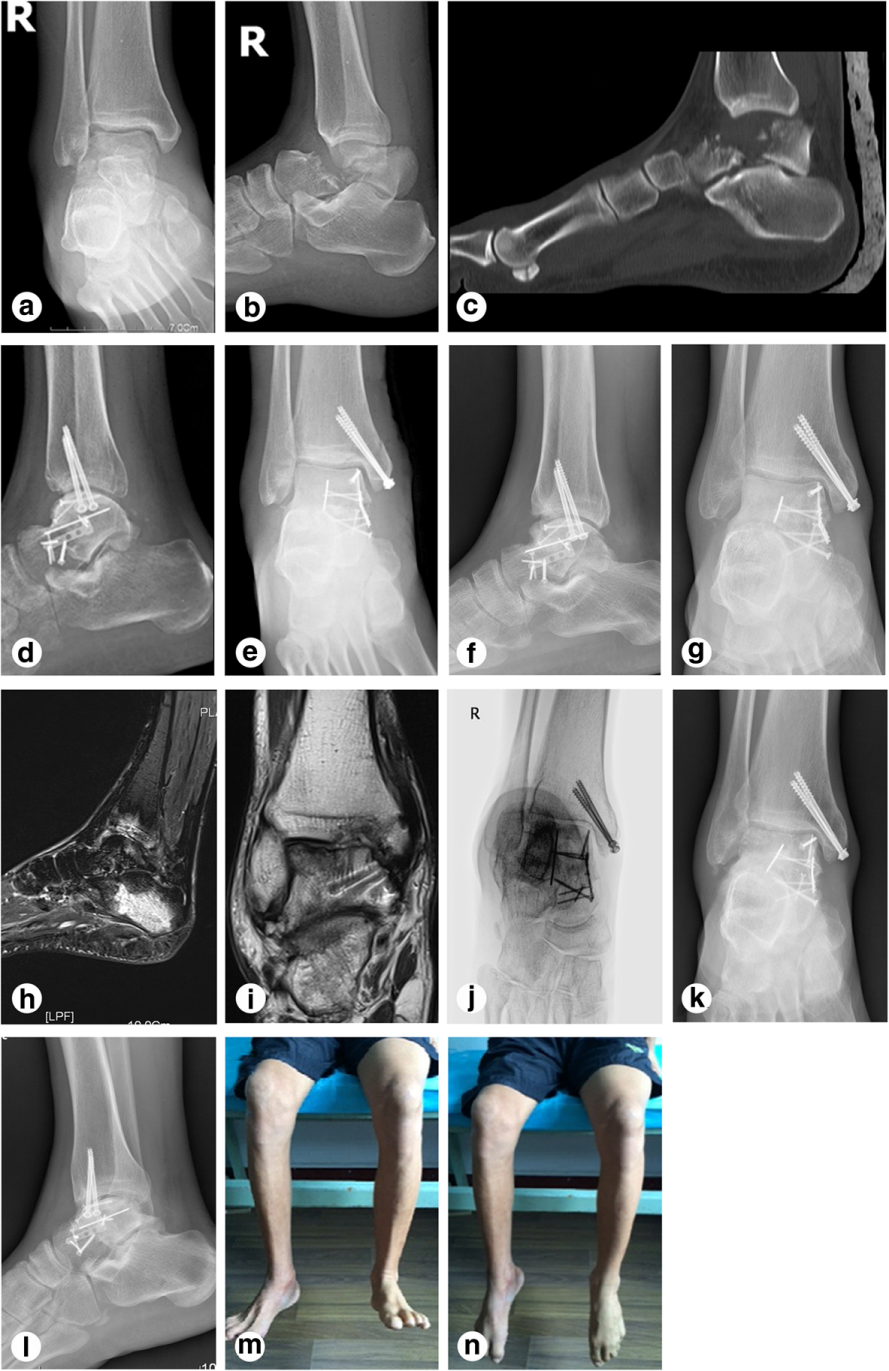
Representative images of case 7 (a 49-year-old male patient with a right ankle injury due to a fall from a 4 m height). a–c X-ray, CT, and 3D reconstruction before operation. d–e Anterioposterior and lateral view of X-ray at 7 days after operation. f–g Anterioposterior and lateral view of X-ray at 6 months follow-up. h–i MRI at 2 years follow-up. Talocrural arthritis and partial AVN of the talar bones were observed. j pronation/oblique (canale) view of X ray at 3 years follow-up. k–l Anterioposterior and lateral view of X-ray at 3 years follow-up. m–n Satisfactory range of ankle motion was achieved at 2 years follow-up
